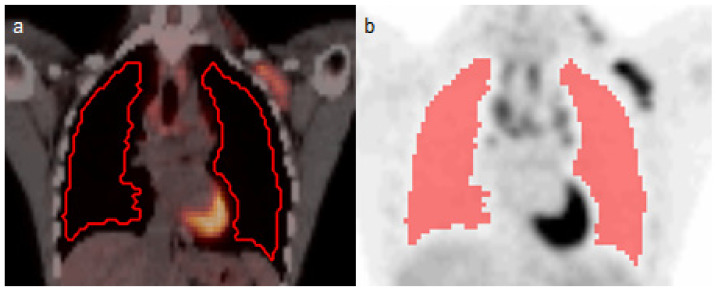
The lung volume of interest (VOI) applied to the (a) CT fused with the PET and (b) PET image.
